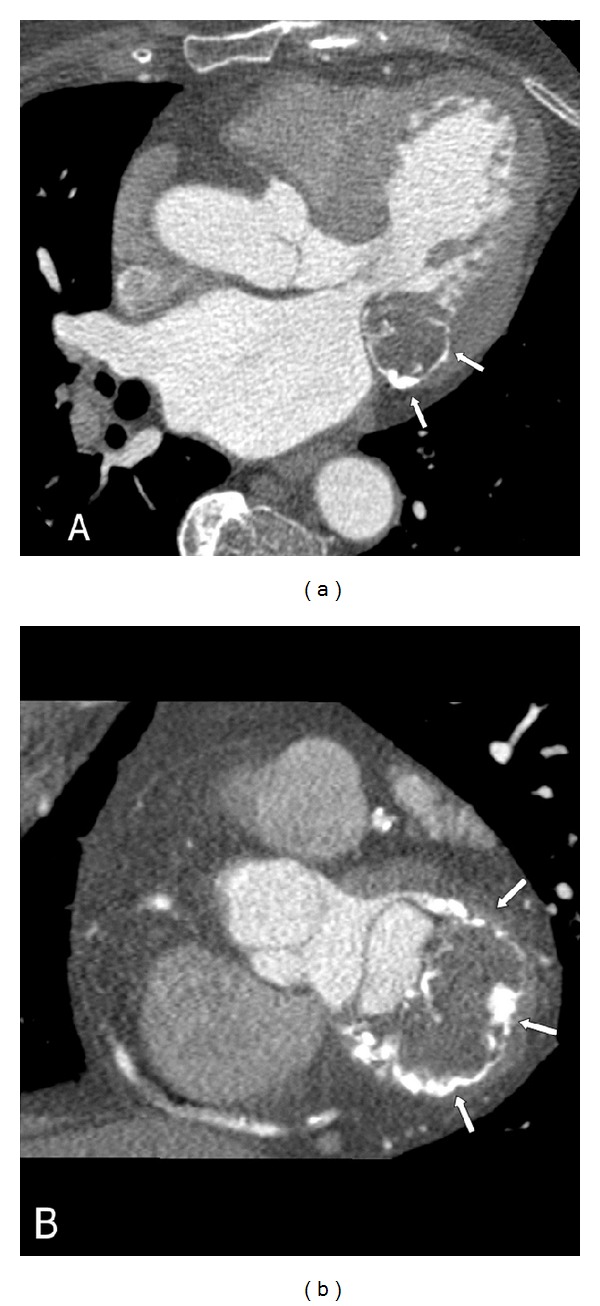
Cardiac CT, long axis (a) and short axis (b) views, showing a crescent-shaped hypodense mass with peripheral linear calcification that produces a shifting of posterior leaflet of mitral valve. No alterations of valvular function were found.
